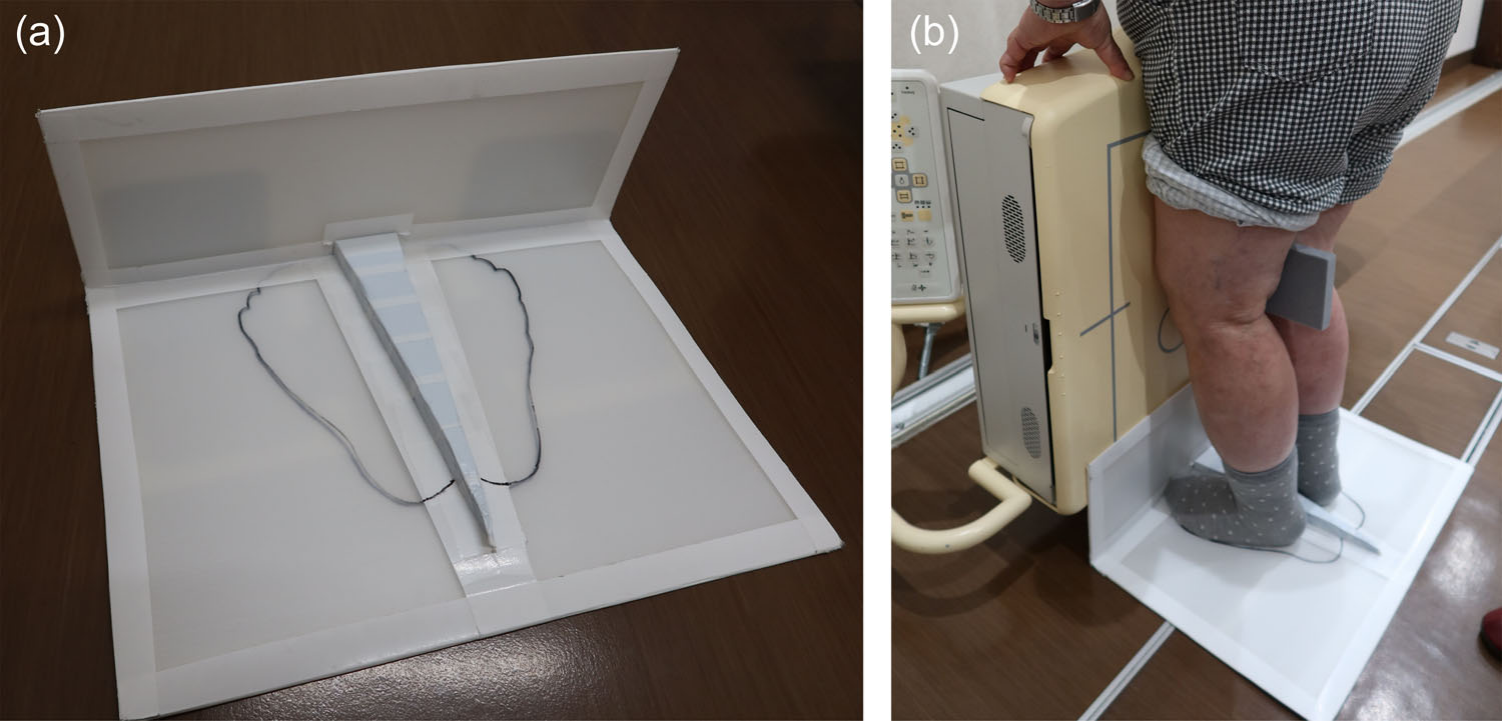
Lower limb fixation device for FFV radiography. (a) The lower limb fixation device is a handmade device for FFV radiography. (b) The patient stands over the device and aligns their toes to the device and the front of their thigh to the radiograph table. FFV, fixed‐flexion view.
